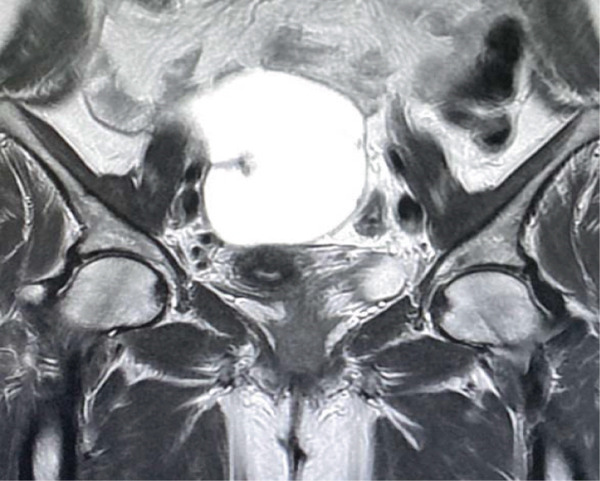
MRI with contrast showed a large, well‐defined right adnexal/ovarian cystic lesion measuring 12 × 10 × 6 cm.
